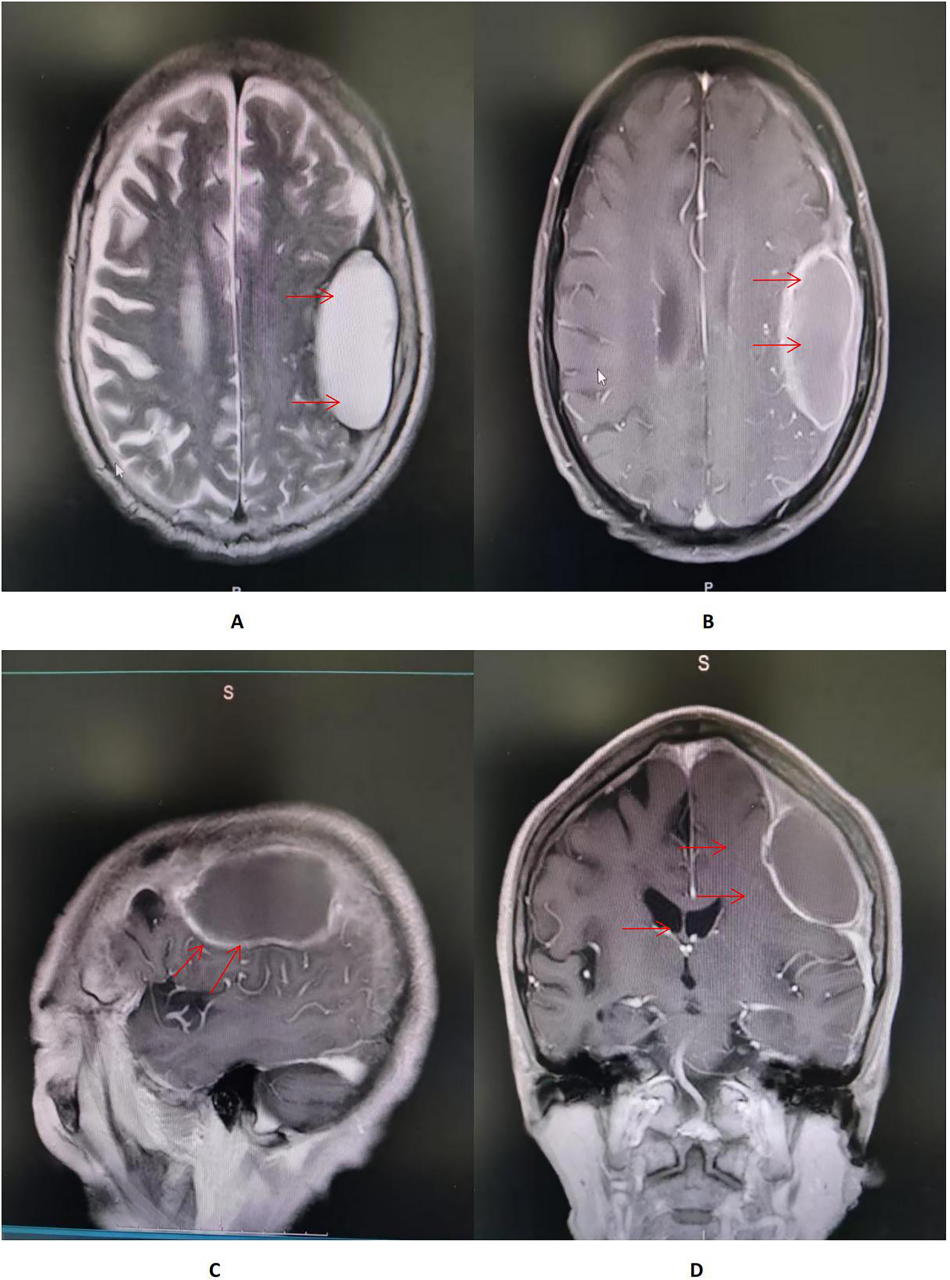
(A,B) Axial T2-weighted/FLAIR and T1-weighted MRI of the head demonstrate a mass-like abnormal signal focus (red arrow) in the left frontoparietal region, measuring approximately 31 × 58 mm. The lesion appeared hyperintense on T2-weighted/FLAIR images and iso- to hypointense on T1-weighted images, situated immediately beneath the inner table of the skull. A moderate perilesional edema was observed, with localized effacement of adjacent sulci. No significant midline shift was identified. (C) Sagittal contrast-enhanced T1-weighted MRI of the head showed an in homogeneously enhancing lesion in the left frontoparietal region, abutting the dura mater with a prominent “dural tail sign” (red arrow). The lesion had well-defined margins, and the surrounding edema showed no enhancement. (D) Coronal contrast-enhanced T1-weighted MRI of the head demonstrated a large mass lesion with rim enhancement and a non-enhancing central area, suggestive of cystic or necrotic components. A significant perilesional edema was present, causing mild mass effect and displacement of the surrounding brain structures (red arrow).
